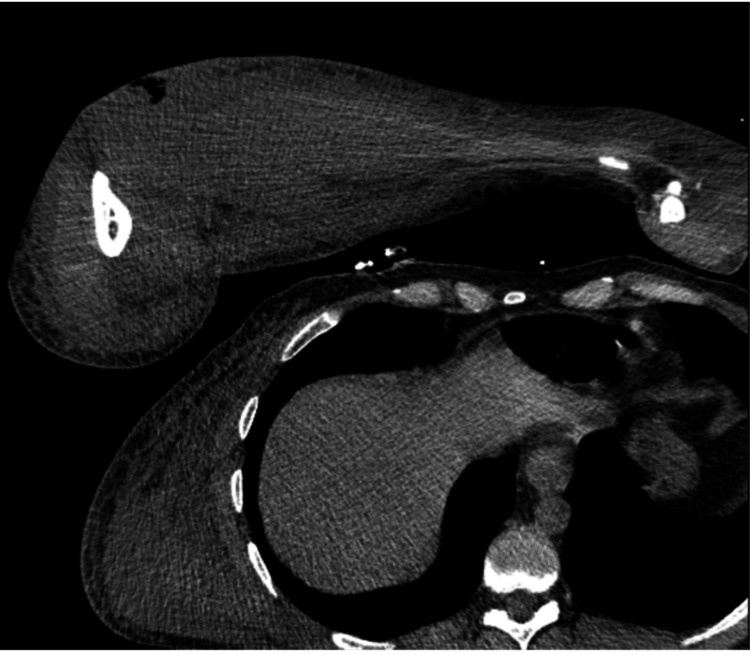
CT of the right upper extremity demonstrating subcutaneous and intramuscular edema of the right forearm with a small focus of gas within the proximal radial aspect of the forearm.
